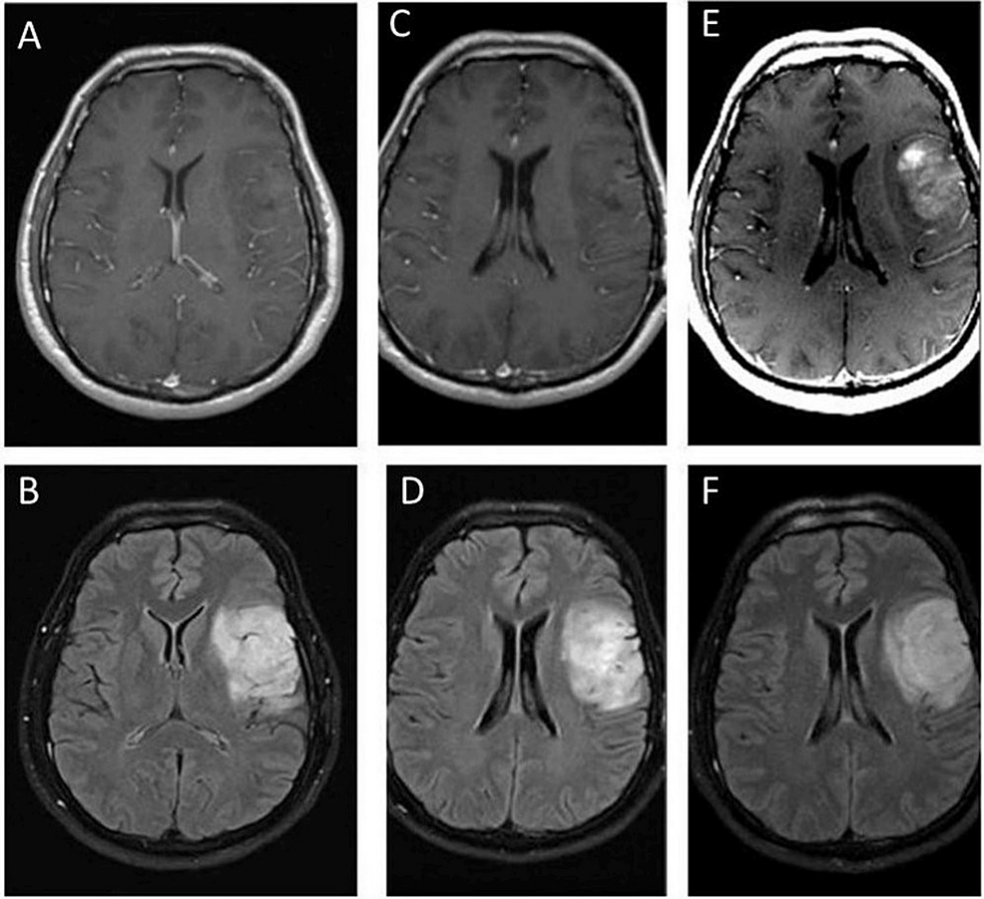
Tumor progression Axial MRI brain. T1 with contrast (top panels) and T2/FLAIR (bottom panels) A-B. Two years after biopsy of the tumor. C-D. Ten months after chemotherapy with PCV. E-F. Tumor enhancement 39 months after chemotherapy with PCV. PCV: Procarbazine, lomustine, and vincristine
